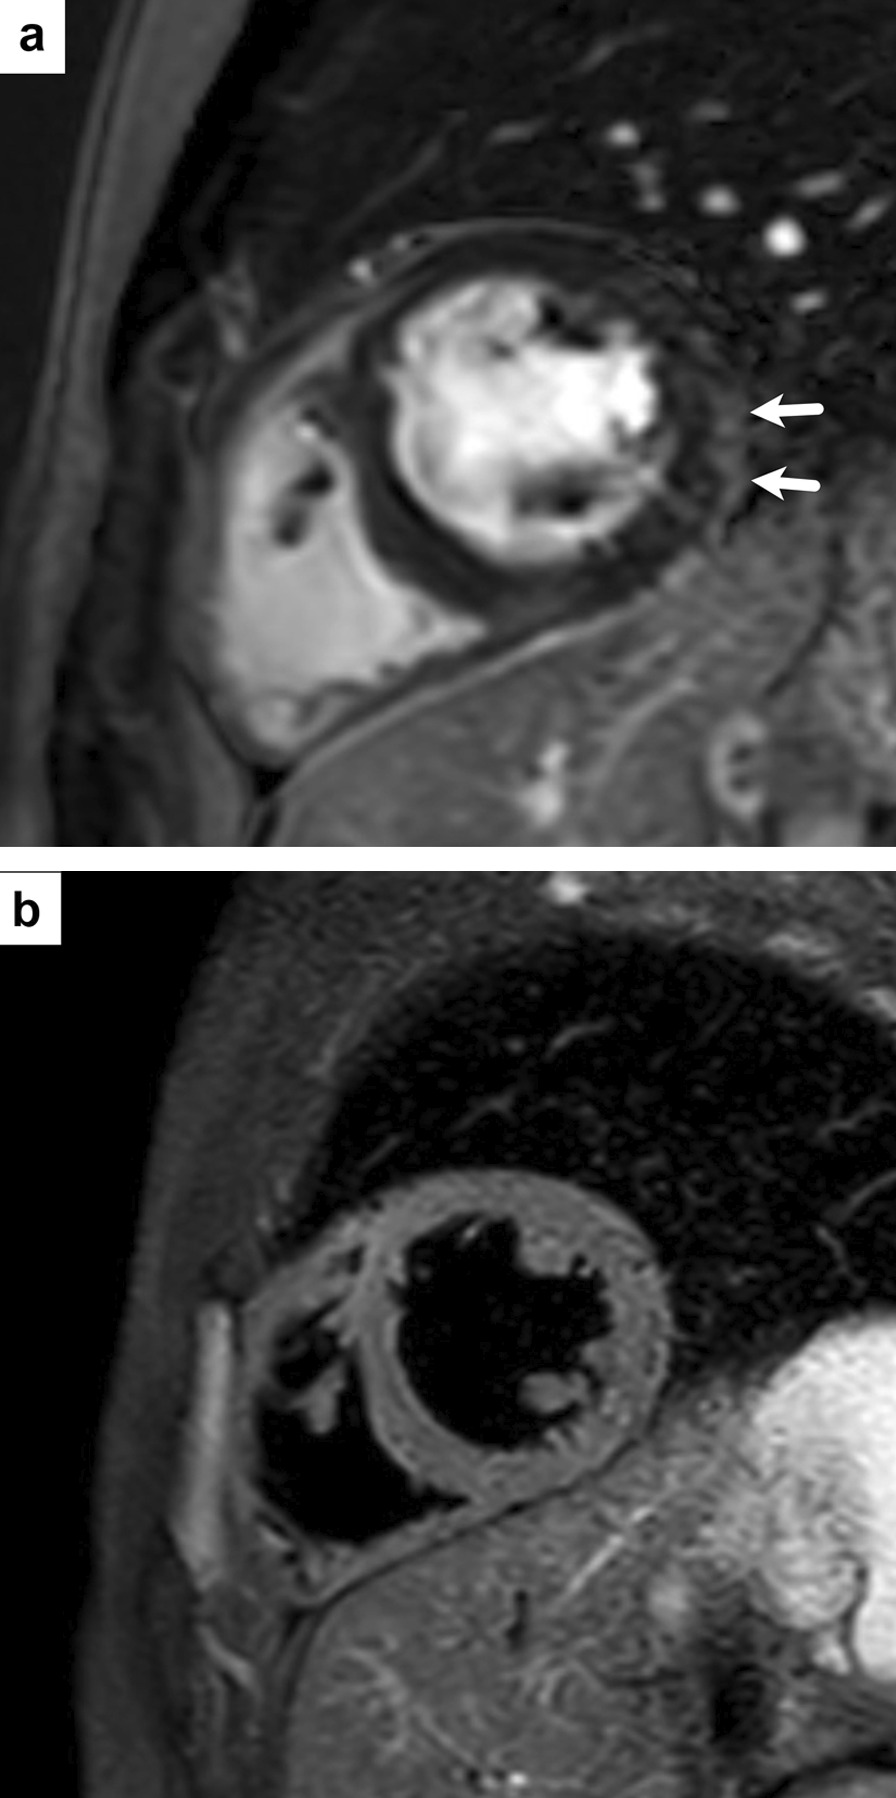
Cardiac MR images obtained four months later revealed that the findings had regressed. a Short-axis late gadolinium-enhanced cardiac magnetic resonance (MR) image with phase-sensitive inversion recovery (PSIR) demonstrate minimal residual subepicardial late gadolinium enhancement at the basal to the mid inferior lateral wall (arrows), and b no myocardial edema on T2-weighted image
